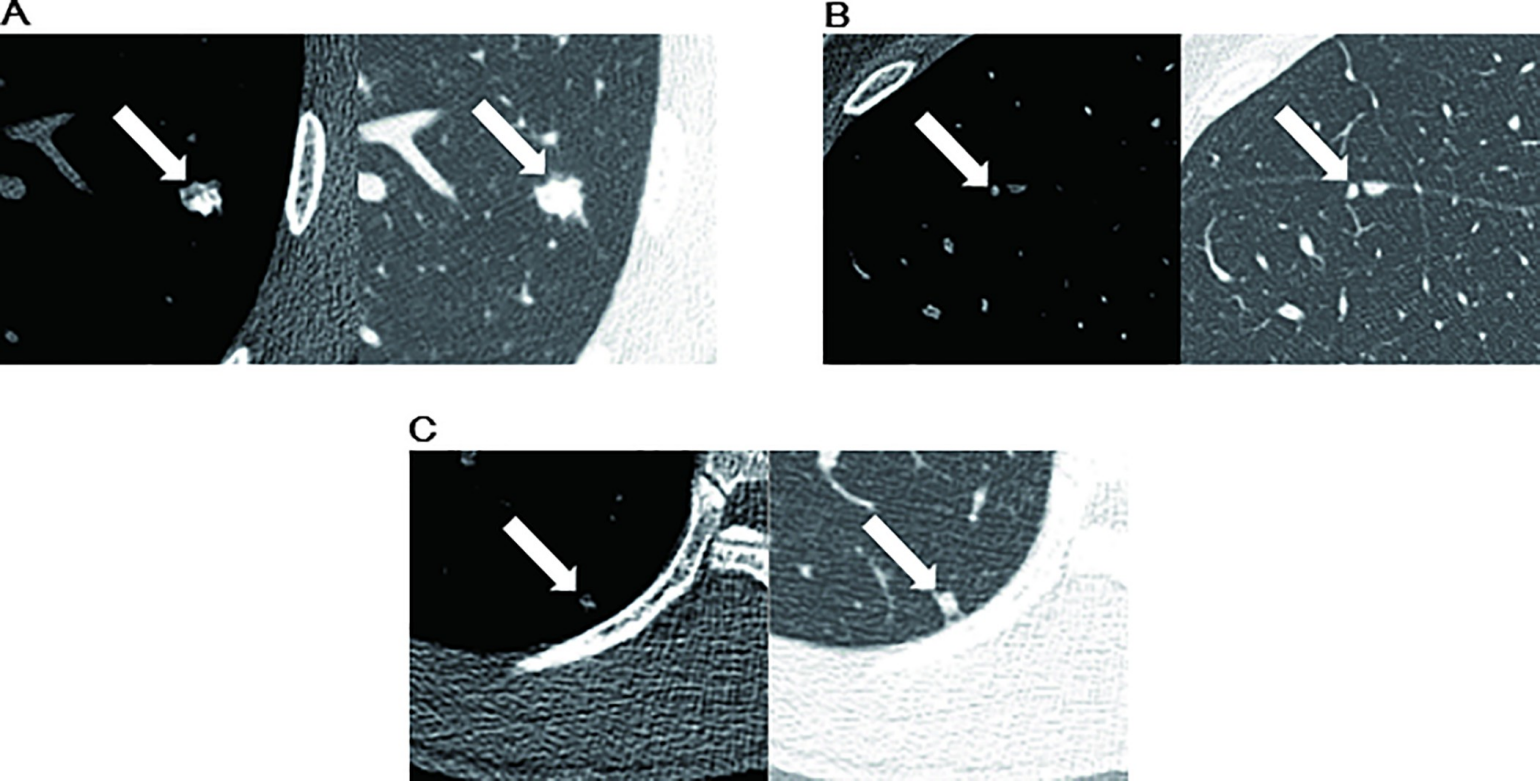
Texture analysis of pulmonary nodules: Metastatic nodules versus non-metastatic nodules. (A) CT scan shows a 12.8-mm solid pulmonary nodule (arrow) with calcification. This nodule shows high mean attenuation (123.6 ± 289.6 HU). The pulmonary nodule was confirmed as a pulmonary metastasis. (B) CT scan shows a 2.9-mm small non-calcified pulmonary nodule (arrow). This nodule shows relatively high mean attenuation (-8.8 ± 255.8 HU). It was confirmed as a pulmonary metastasis. (C) CT scan shows a 2.4-mm small non-calcified pulmonary nodule (arrow). This nodule has relatively low mean attenuation (-229.9 ± 212.3 HU). It was confirmed as an intrapulmonary lymph node.
